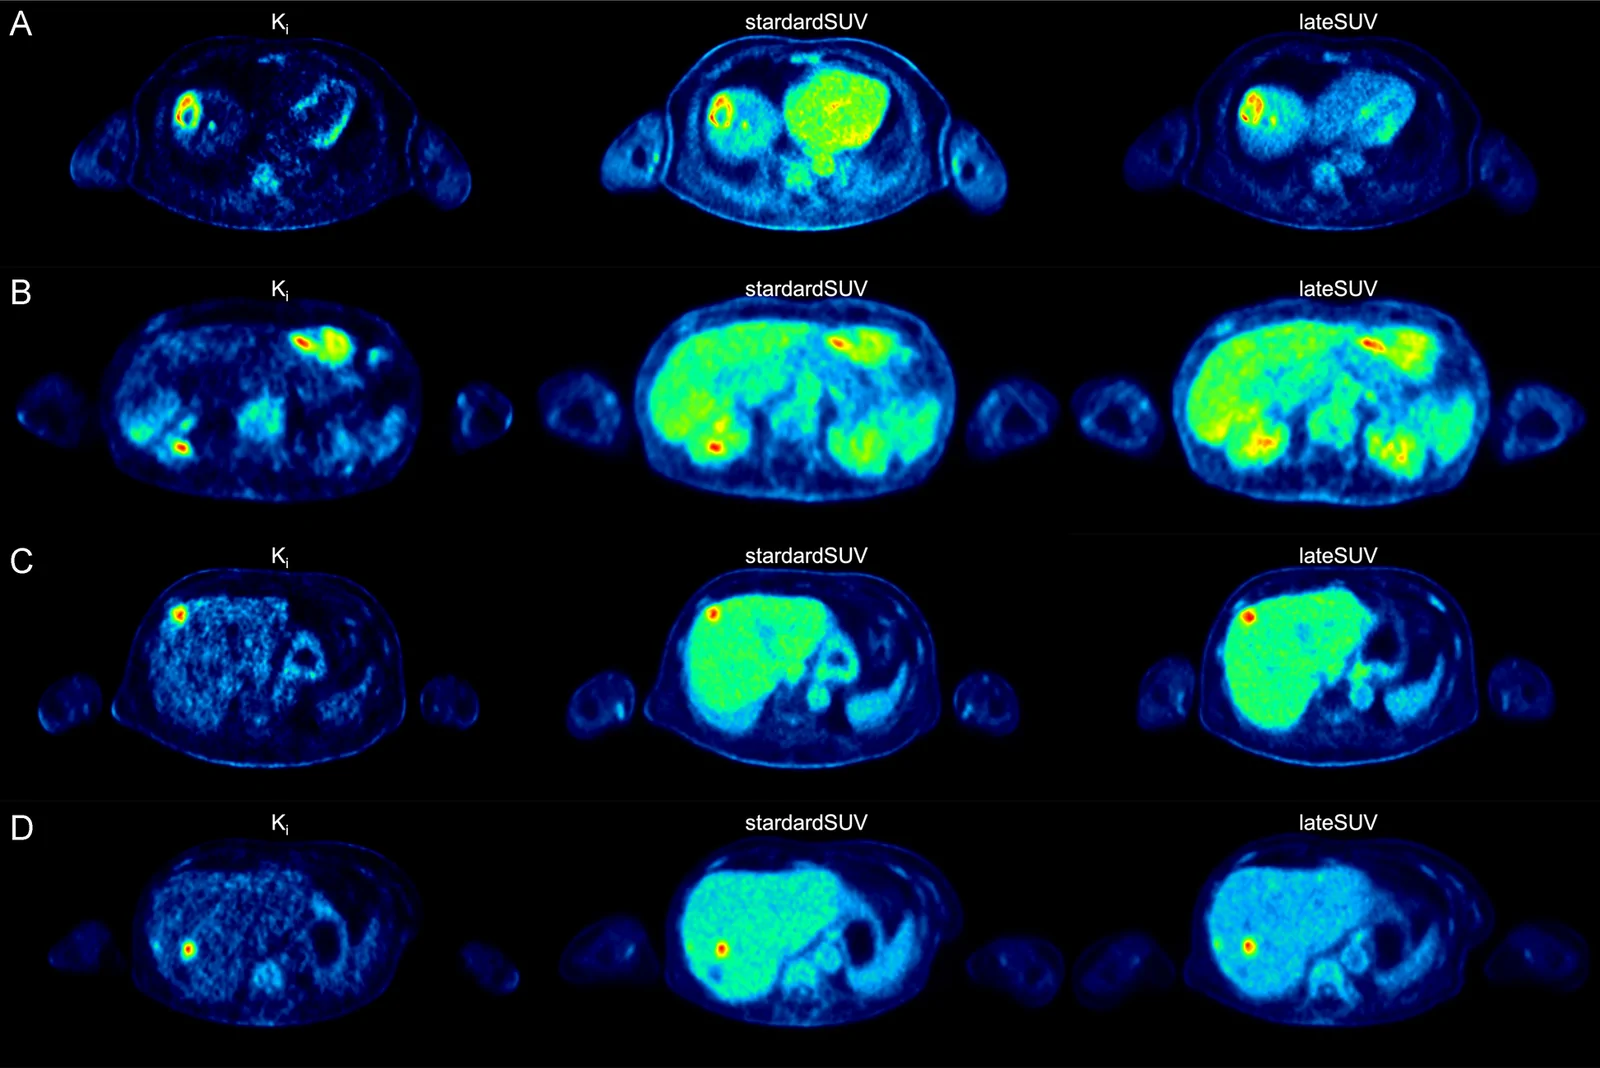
In patients No. 3, 6, 10, and 14 (rows A–D), Patlak K_i_ images demonstrated markedly higher lesion contrast for liver metastases compared with static images, whereas late SUV static images provided superior contrast compared to standard SUV static images
